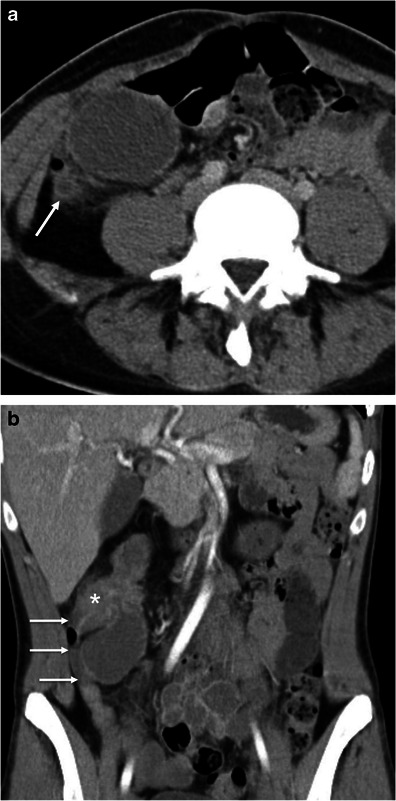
Acute appendicitis. Axial (a) and coronal (b) contrast-enhanced CT scan shows the retrocaecal enlarged fluid-filled appendix (*arrows*) associated with adjacent fat stranding and reactive wall thickening of the ascending colon (*asterisk*)
